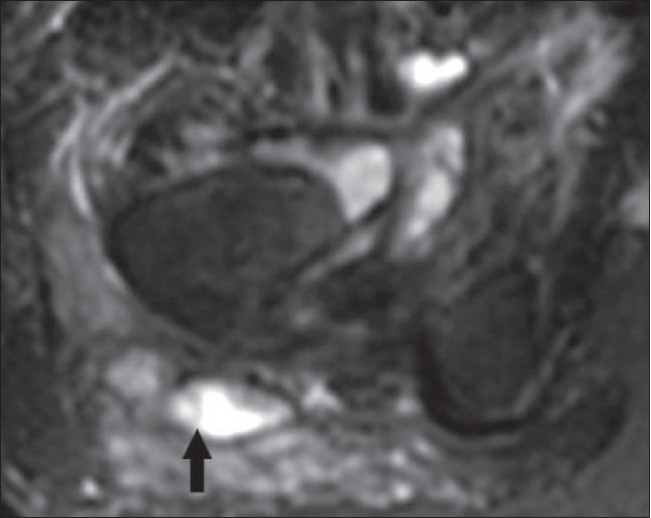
Tuberculous bursitis of the right trochanteric bursa (arrow) on an axial, fat-suppressed T2W MRI. The distended bursa contains fluid, whereas the thickened synovium is of relatively low signal intensity
